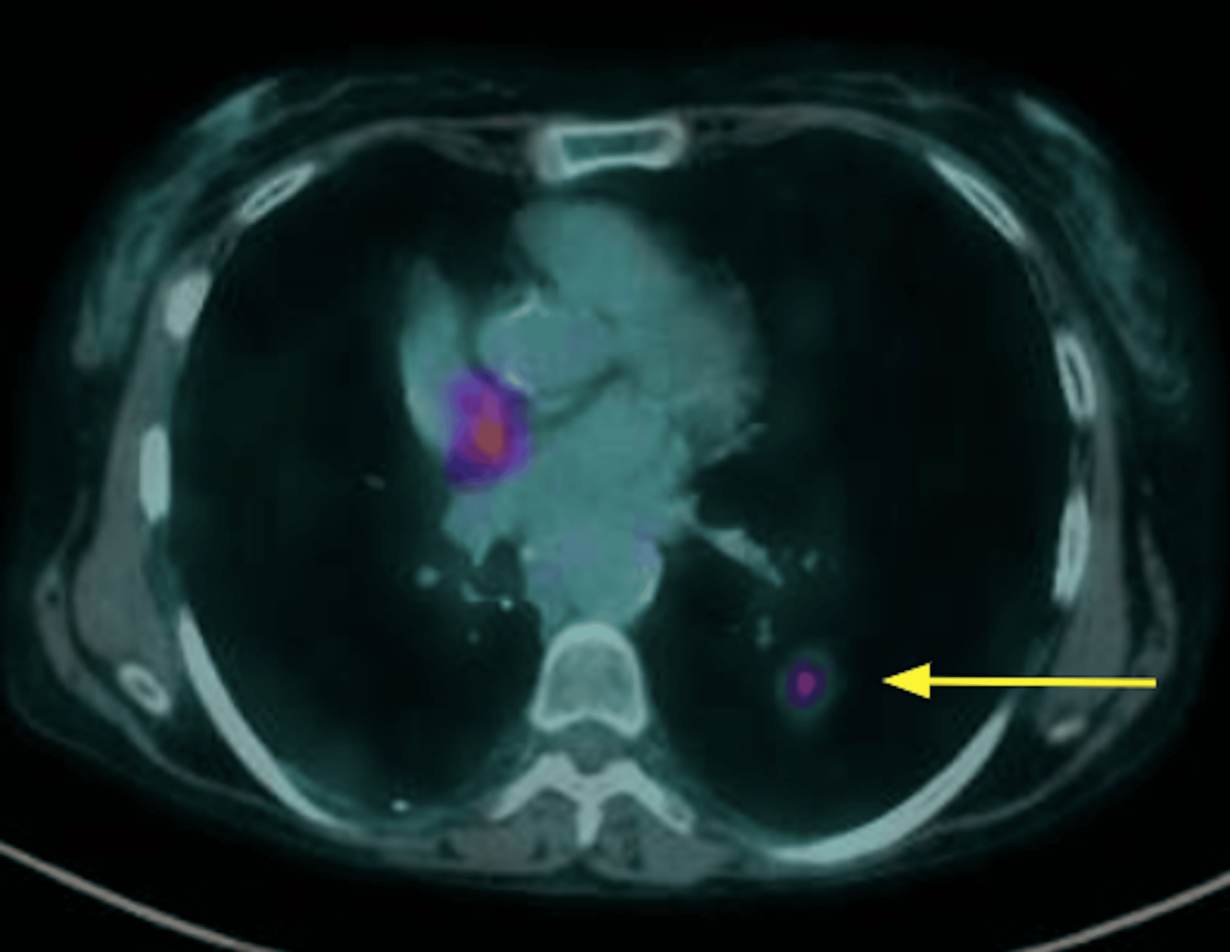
PET-CT showing progressive pulmonary disease (arrow) PET-CT: positron emission tomography, CT: computed tomography
